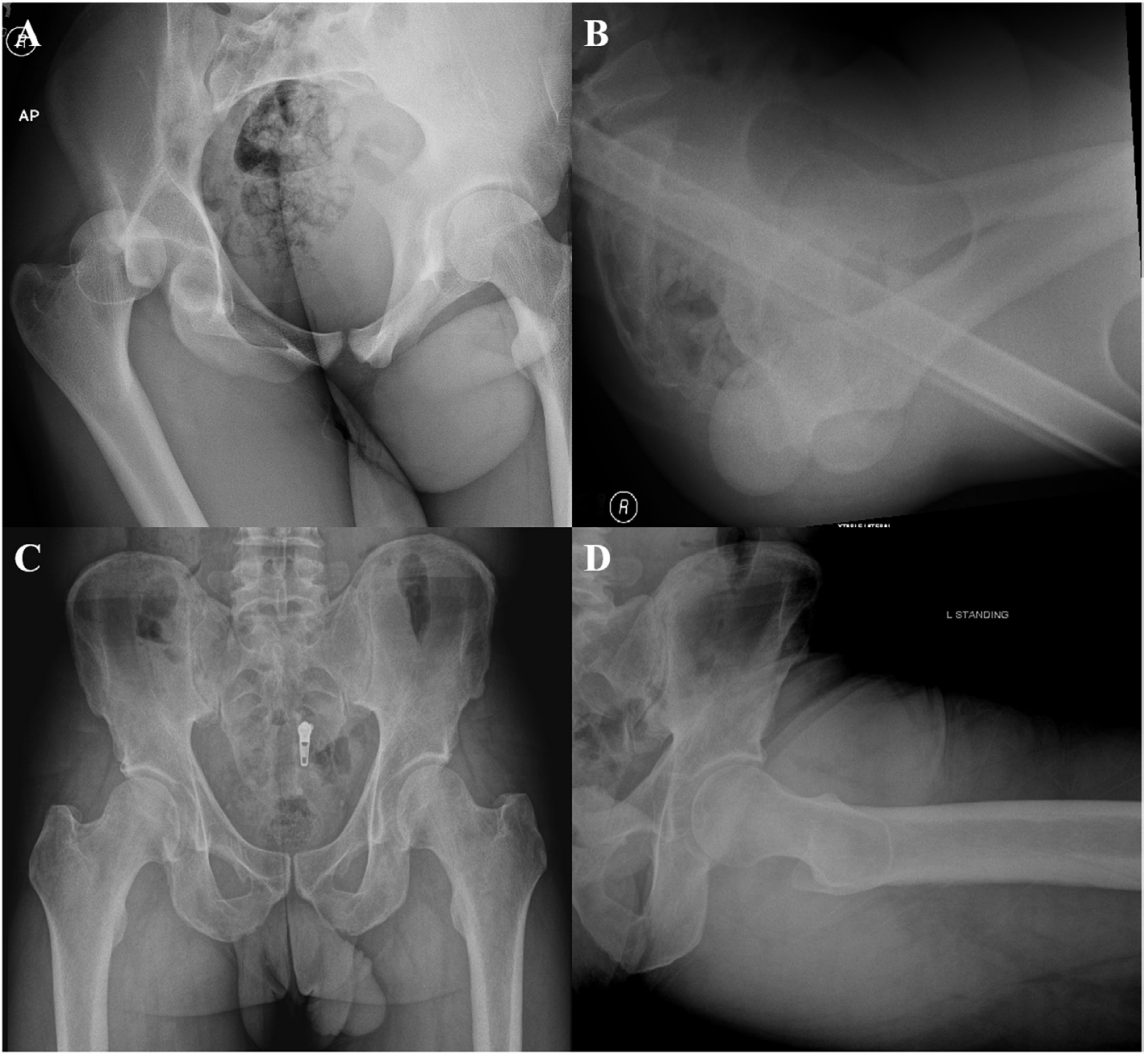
Anteroposterior (A) and cross table (B) radiographs demonstrating a posterior hip dislocation. Anteroposterior (C) and Dunn (D) views demonstrating a borderline dysplastic patient (LCEA 21.6) with a cam deformity (AA 63).
